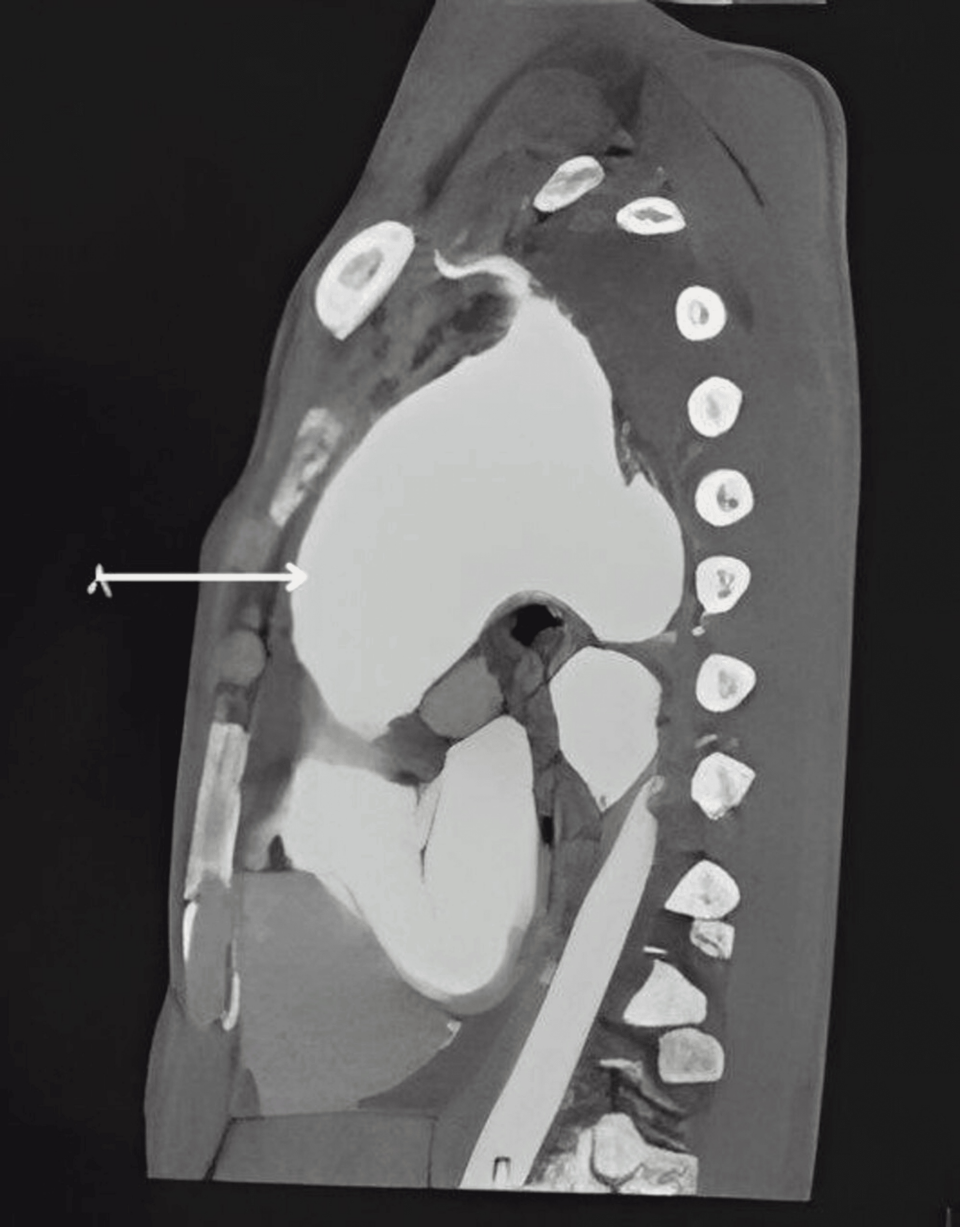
Sagittal chest computed tomography showing a large aortic aneurysm involving the ascending aorta, arch of the aorta, and proximal part of the descending aorta.
